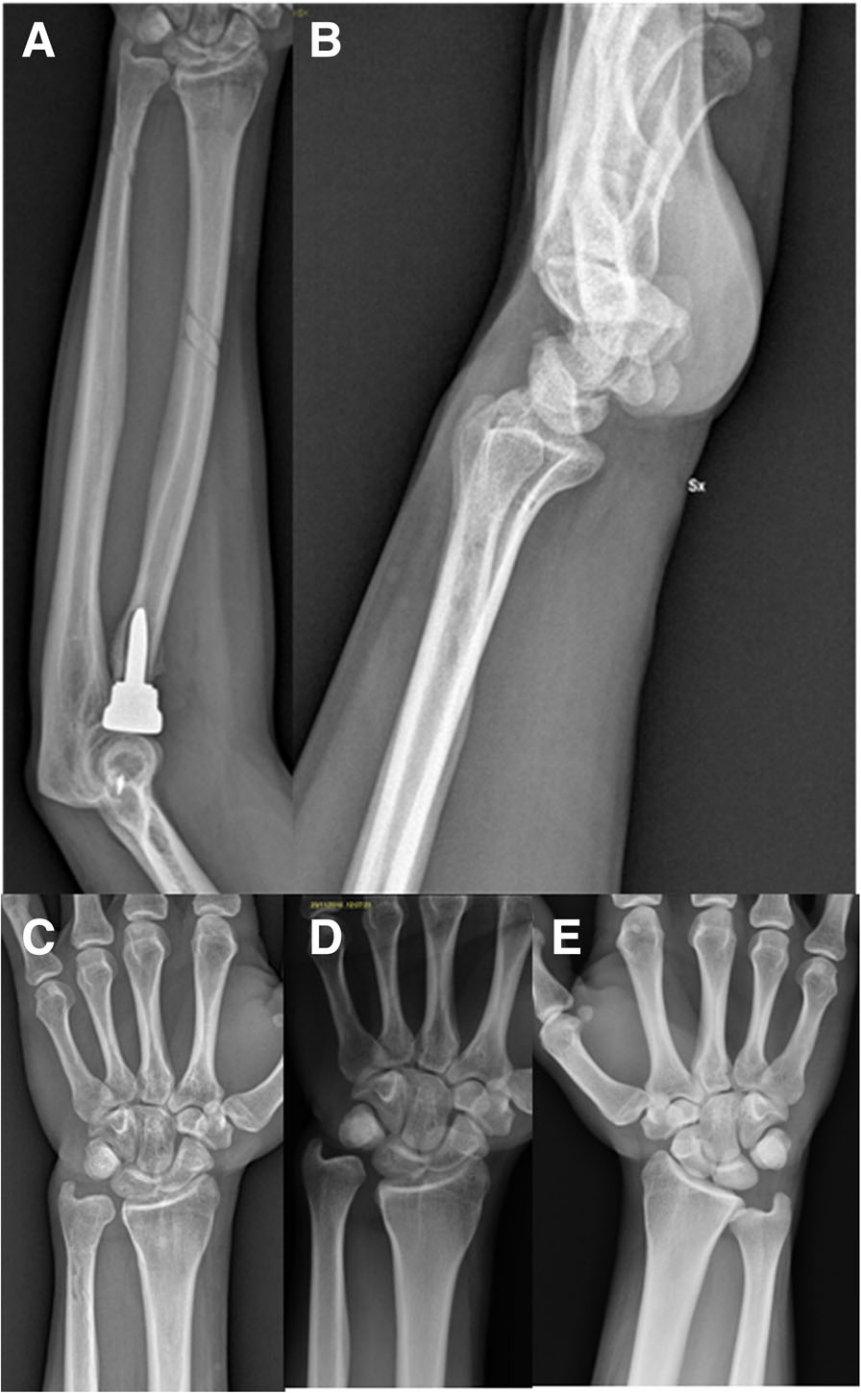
1 year X-rays, clinical case 3. Follow up X-rays at 1 year of follow up, showing the radial head prosthesis in situ and the whole forearm (a). The lateral view shows no dorsal dislocation of the distal ulna (b). At the DRUJ a slight recurrence of the ulnar plus is evident (c), even if non symptomatic. Nevertheless the improvement pre-operative wrist x-ray (d) is evident. e Image shows the opposite side normal wrist
